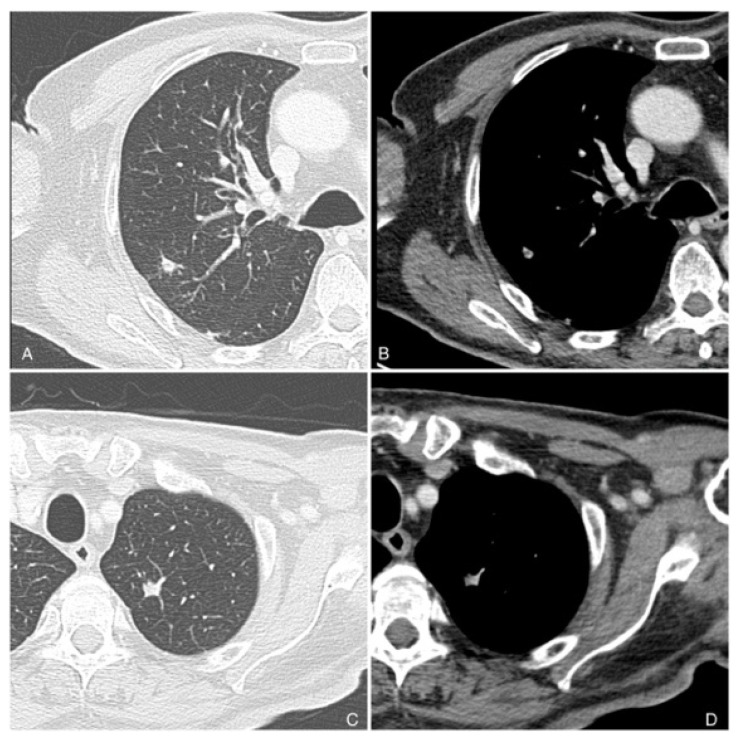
Chest CT images (A–D) of a 80-year-old male with squamous carcinoma of the internal canthus of the left orbit, shows two part-solid nodules, one found in the apical segment of the left upper lobe of the lung (C,D), and the other noted in the superior segment of the right upper lobe of the lung (A,B) were classified as intrapulmonary metastasis. Multidetector computer tomography of the abdomen and pelvis was performed with and without contrast. The examination was performed in the four-phase protocol, before and after contrast administration (Iomeron 370–400 Bracco, Milan, Italy, injected at 3.5/4 mL/s): unenhanced, arterial, portal venous and delayed phases, using a Dual Source CT (Siemens Somatom^®^, Siemens Healthcare, Forcheim, Germany).
